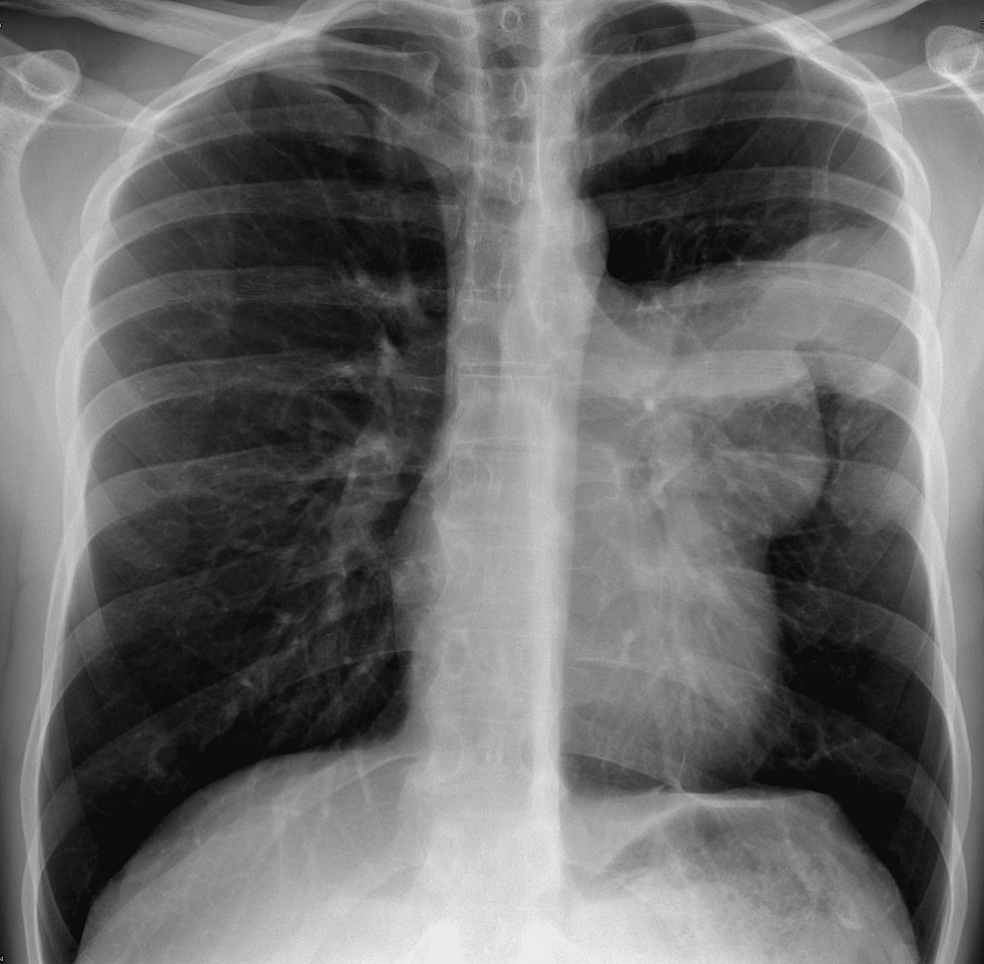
Chest X-ray showing a well-marginated mass in the left perihilar region.
